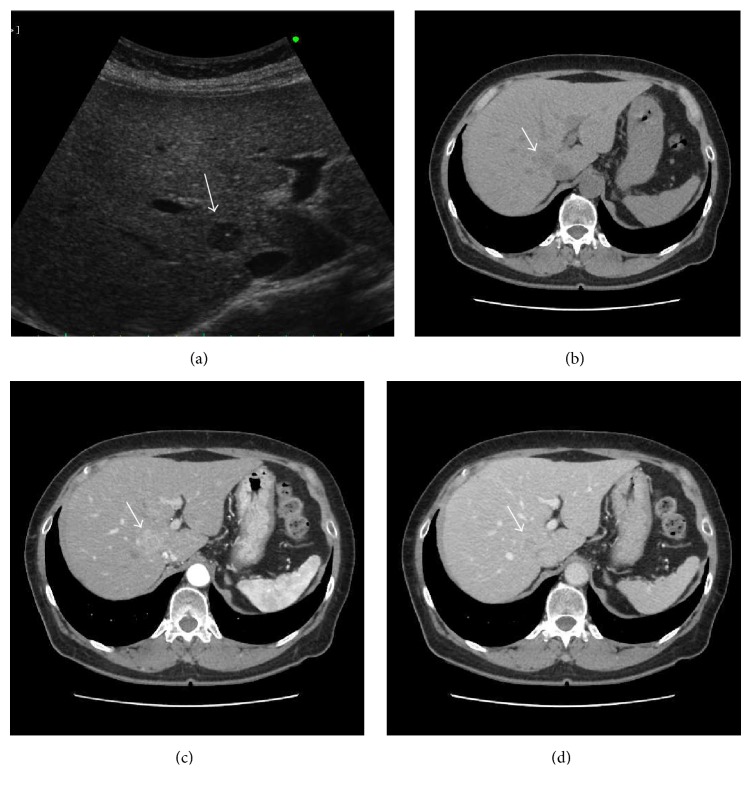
Ultrasonography and computed tomography. US revealed a 14 × 10 mm well-defined, hypoechoic mass (arrow) in the posterosuperior subsegment of the right hepatic lobe (segment 7) (a). Plain CT shows a low-density mass at the right hepatic lobe (arrow) (b). The tumor was enhanced in the arterial phase (c) and washed out in the portal phase (d).
